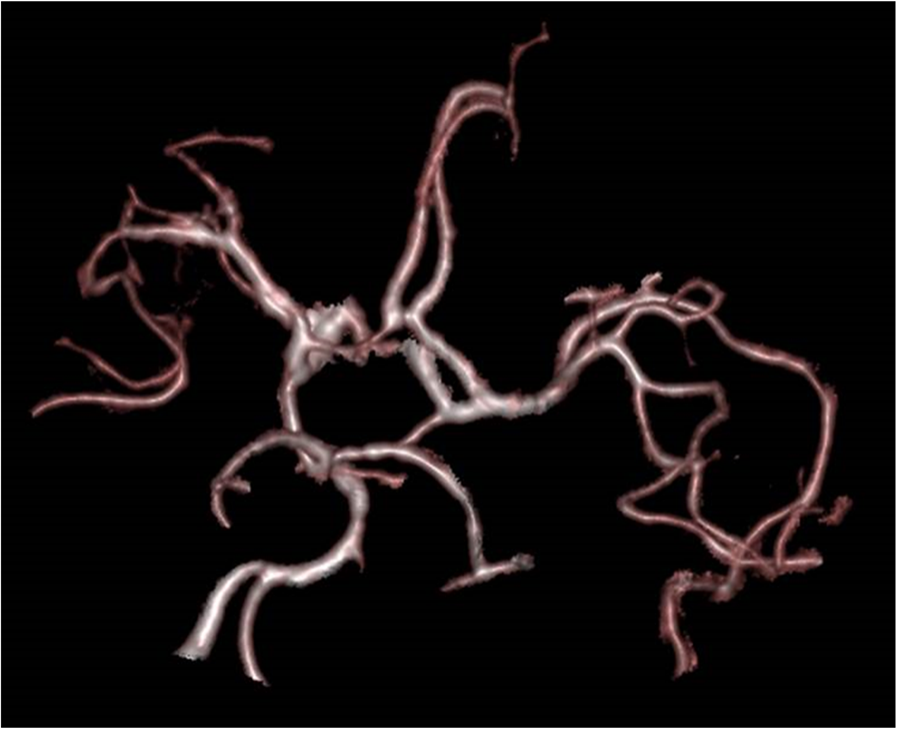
Magnetic resonance imaging vascular volume rendering reconstruction obtained from the 3D-T1 gadolinium sequences. Absence of proximal vascular stenosis nor thrombosis
